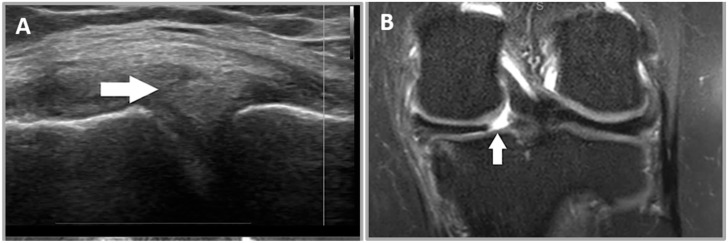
A 45-year-old female patient who presented with left knee joint twisting injury and medial sided knee pain. (A) US demonstrated extruded medial meniscus, which protrudes beyond the medial tibiofemoral joint line. (B) A coronal proton density MRI image demonstrating a full thickness radial tear of the posterior root attachment (white arrow) with medial meniscal extrusion.
